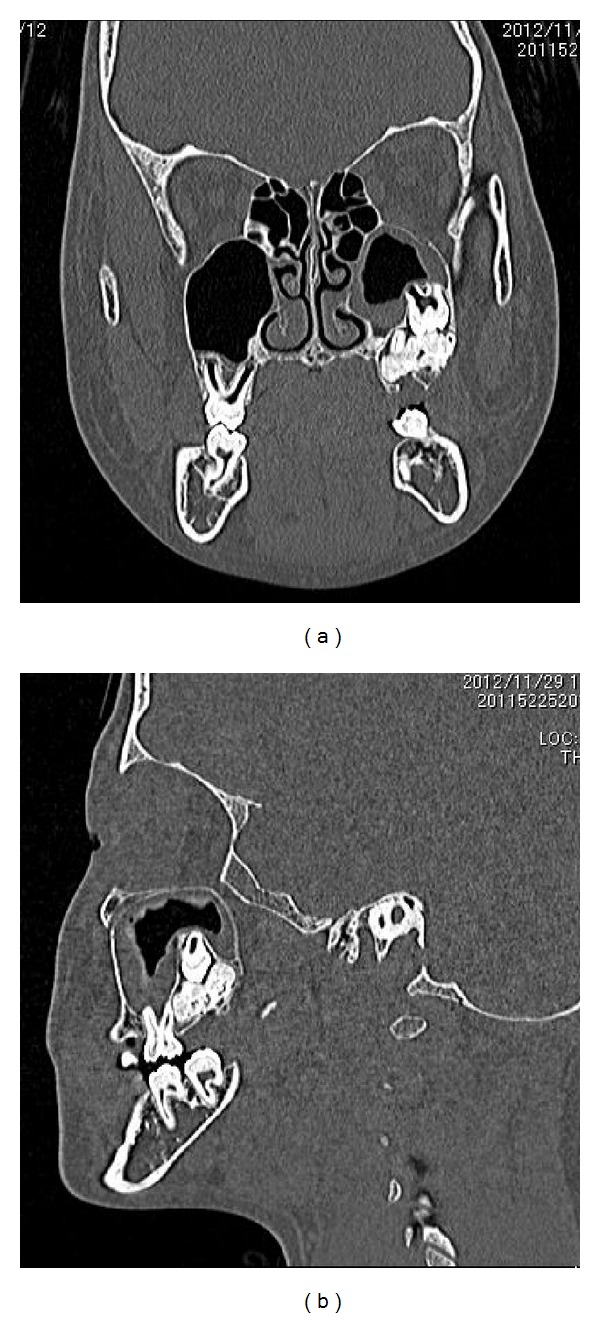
CT view of the lesion. (a) Coronal view. (b) Sagittal view.
